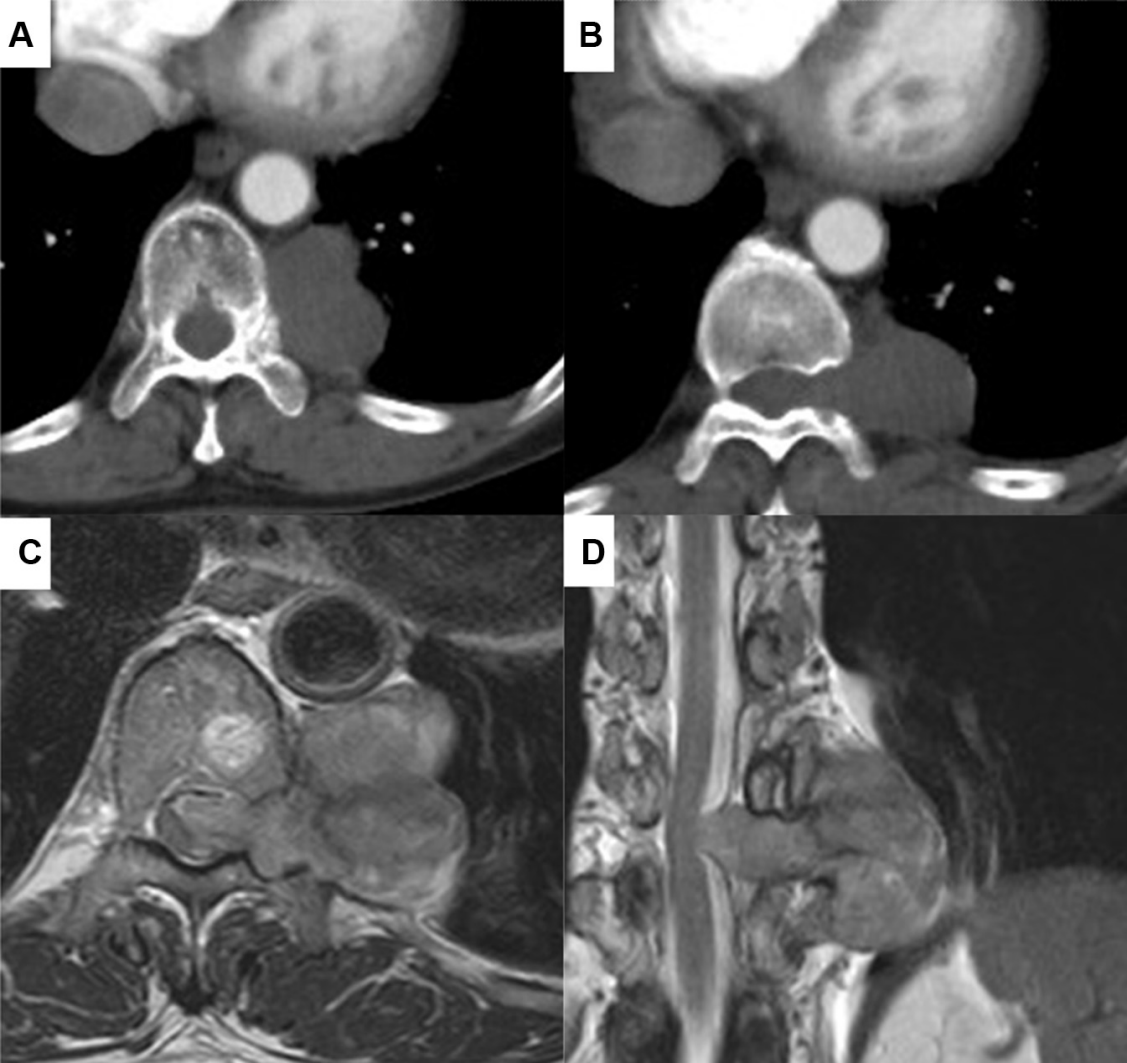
A and B, Computed tomography of the chest images in the axial plane. Magnetic resonance imaging in the axial view (C) and coronal view (D).
